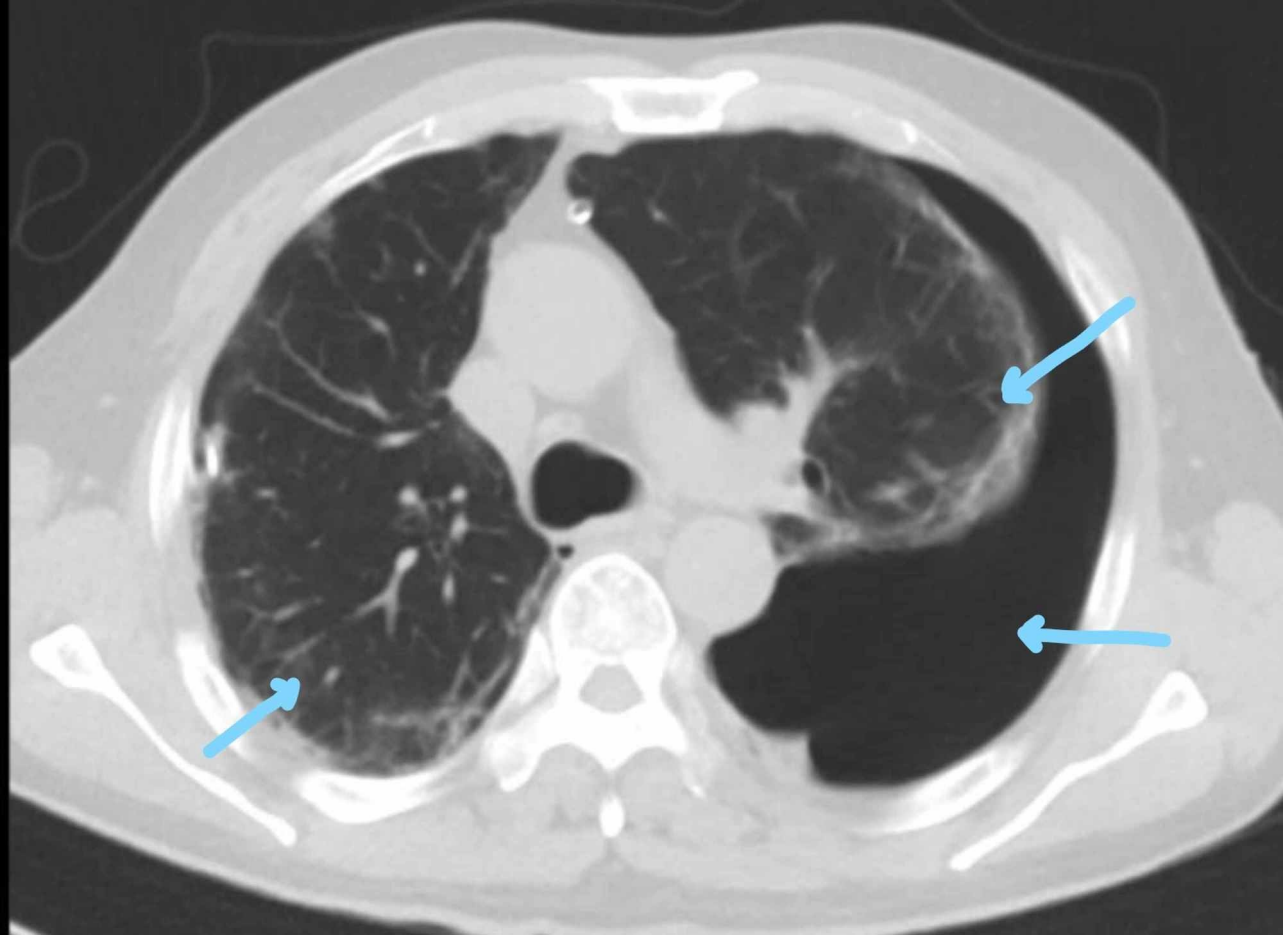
CT of the thorax demonstrating bilateral emphysematous bullous changes with pneumothorax
